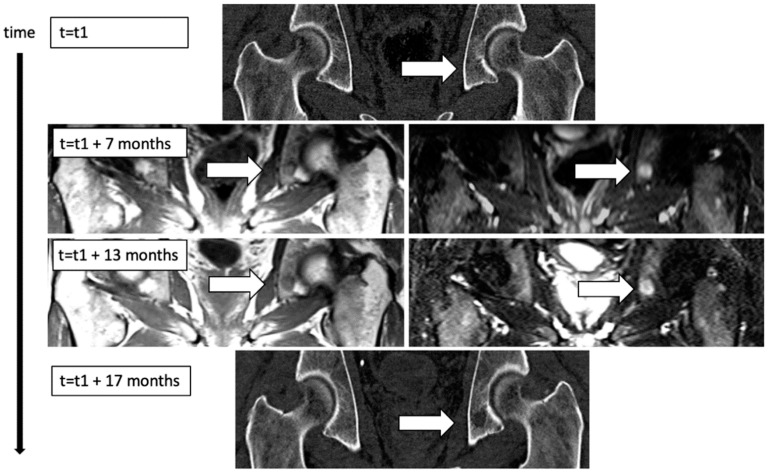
Development of a focal lesion in MRI over time with appearance of a new, corresponding osteolytic lesion in computed tomography (CT) imaging. At initial CT-imaging (t1), trabecular Scheme 1219. mm^3^, upper left (T1w tse) and right (T2w stir)), which then showed a three-dimensional growth dynamic of 3.37 mm^3^/day for 6 months to reach a volume of 1833 mm^3^ (t_2_ = 13 months, lower left and right). Four months later, the next CT imaging was performed and revealed an osteolytic lesion (t_3_ = 17 months, volume = 1022 mm^3^, lower image) in the corresponding position.
